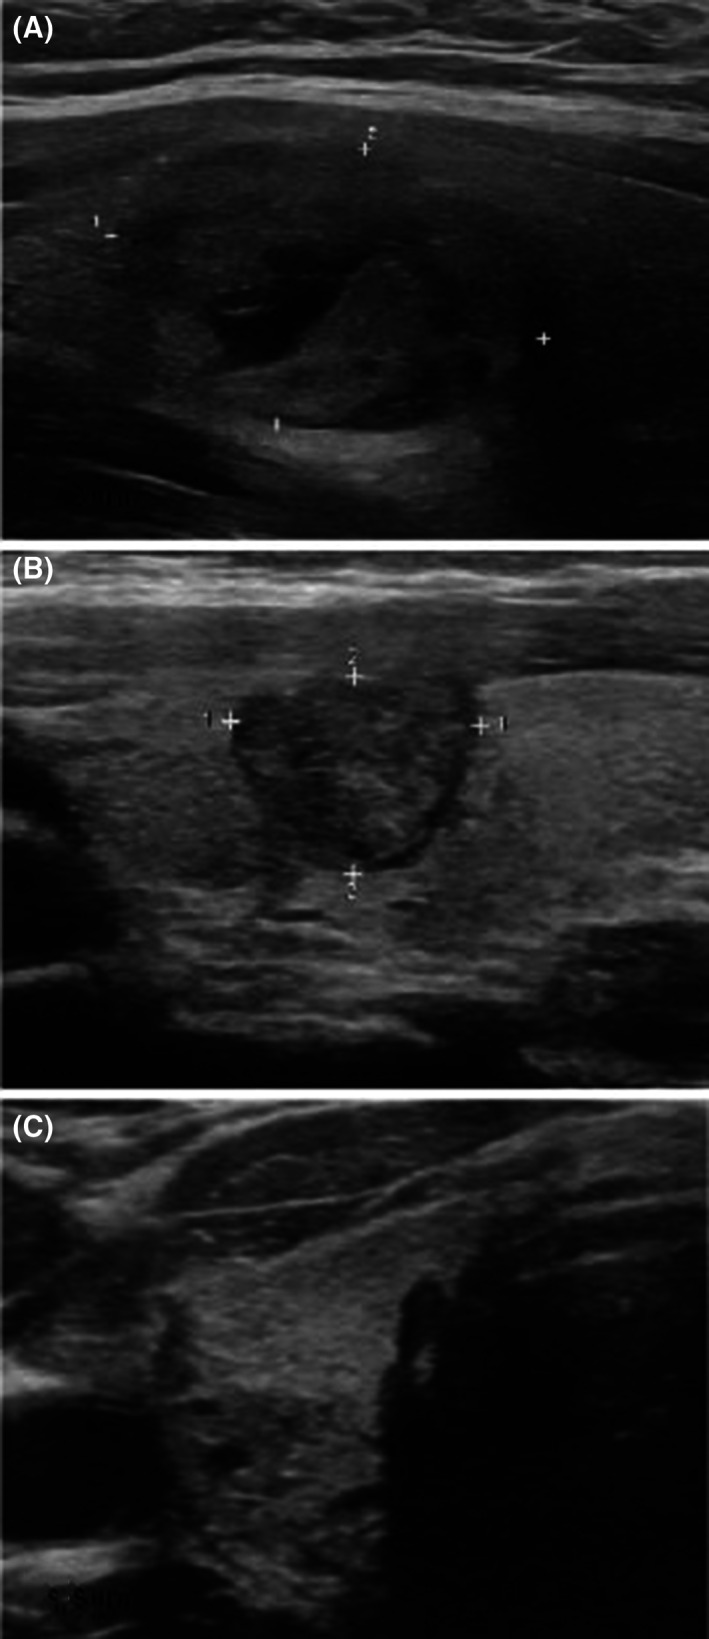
Preoperative (A) ultrasound of a thyroid nodule compared with a postoperative (B) ultrasound at 3 months after RFA for one patient, showing decrease in size. In contrast, postoperative ultrasound 2 years after RFA (C) for another patient that shows loss of a distinct nodule border.
